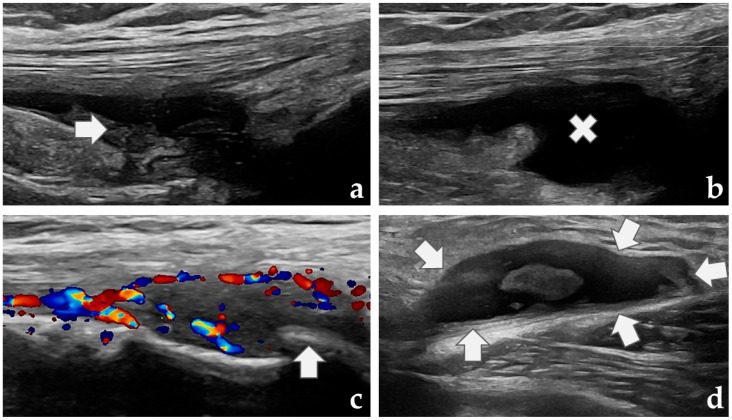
Ultrasound biomarkers of synovitis: (a) suprapatellar recess with severe synovial hypertrophy (arrow); (b) suprapatellar recess with major effusion (cross); (c) lateral parapatellar recess with severe Doppler activity (color) and a moderate osteophyte (arrow); (d) Baker’s cyst (arrows) with synovial hypertrophy.
